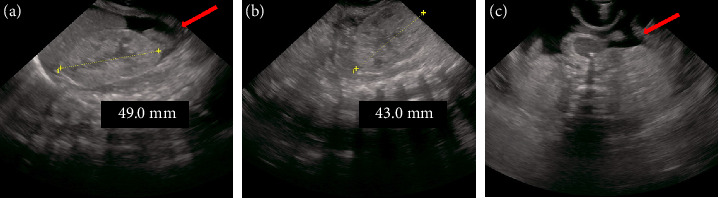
Abdominal ultrasound image at the time of diagnosis of CNS. No difference exists in the size of the kidneys ((a) right kidney, (b) left kidney). No hypoplasia or cystic kidney was observed. Ascitic fluid was observed (a, c).
